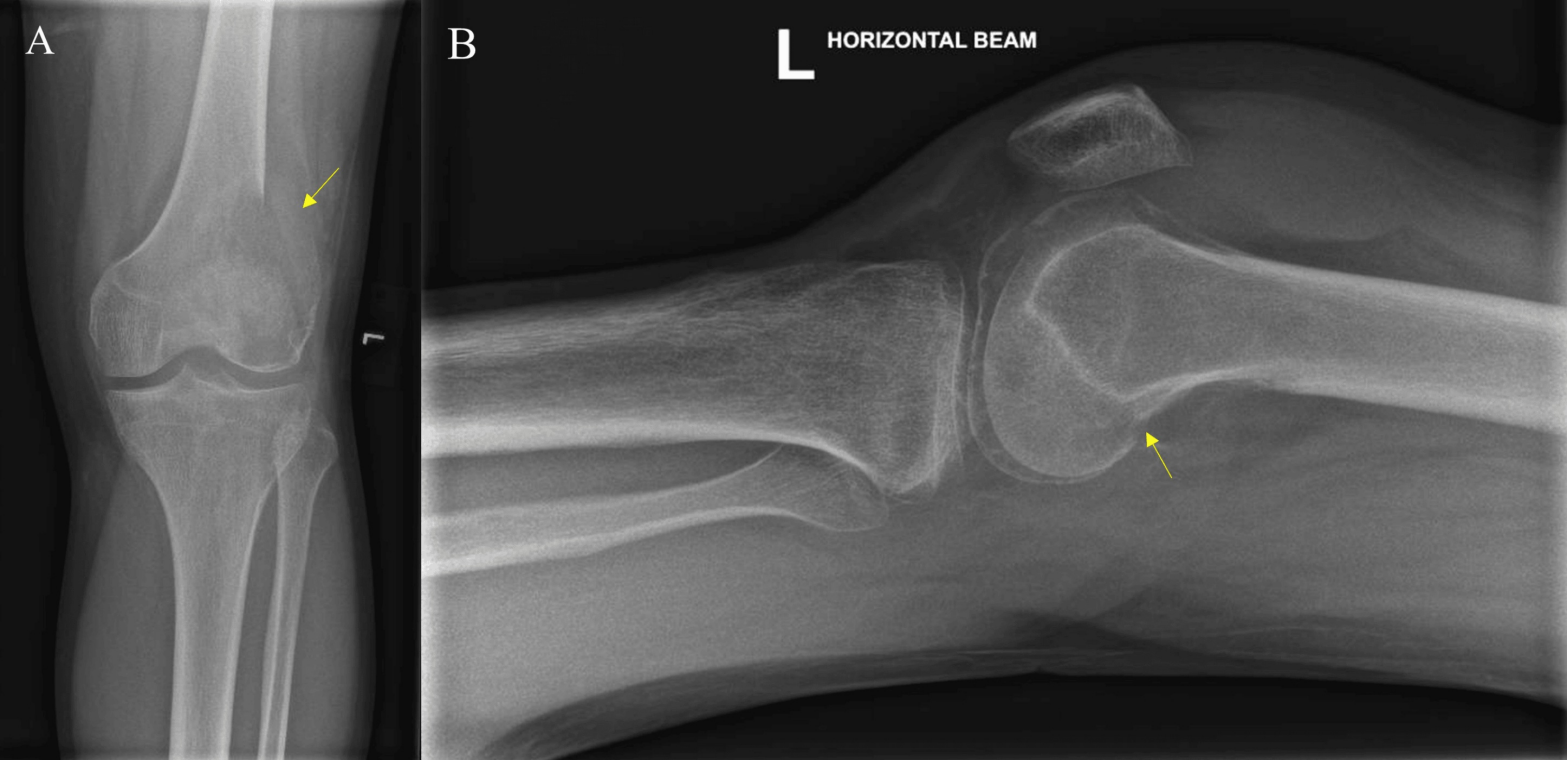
Plain radiographs of the left knee (A) Anteroposterior view identifying a lytic lesion and cortical irregularity over the lateral femoral condyle; (B) lateral view showing a lytic lesion in the distal femur and cortical irregularity over the posterior aspect
